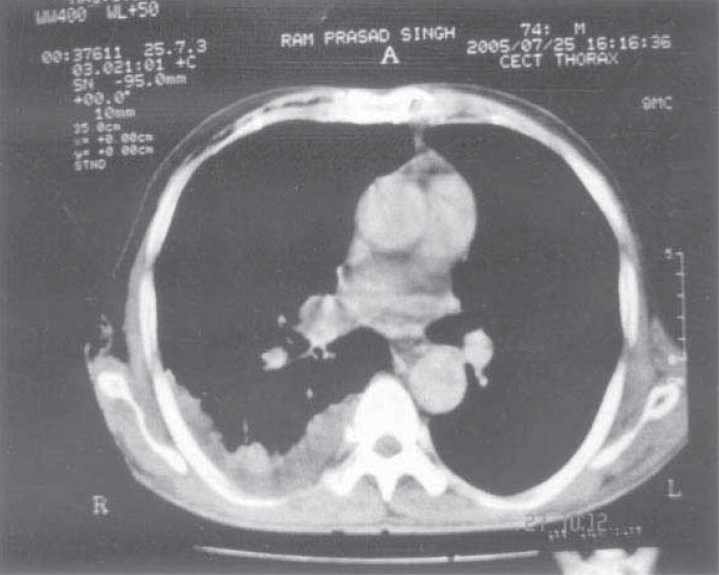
CT thorax showing right sided pleural effusion.
